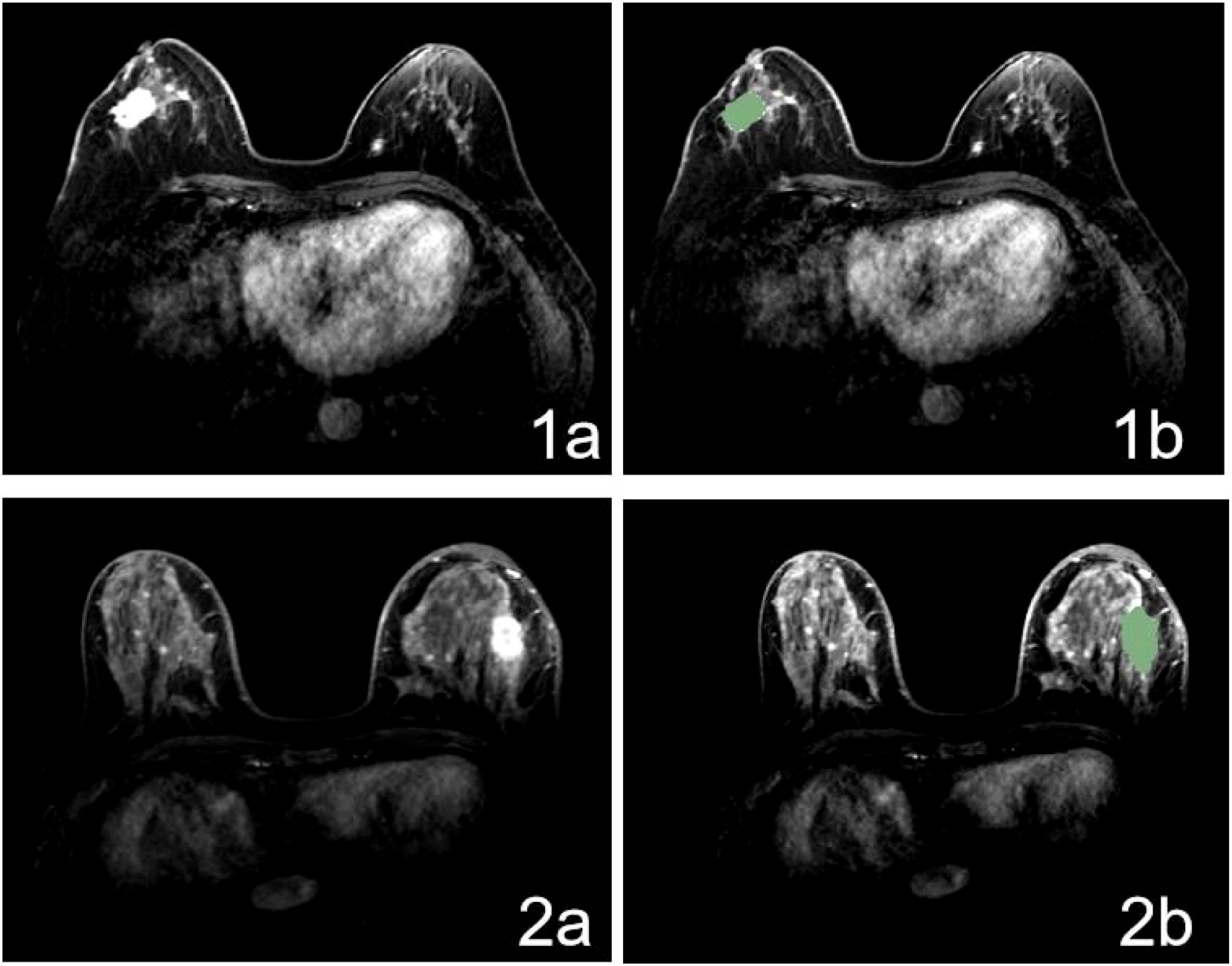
MR Image for patient with positive (1a) and negative (2a) IMLN and ROI delineating based on DCE-MR image (1b, 2b).
